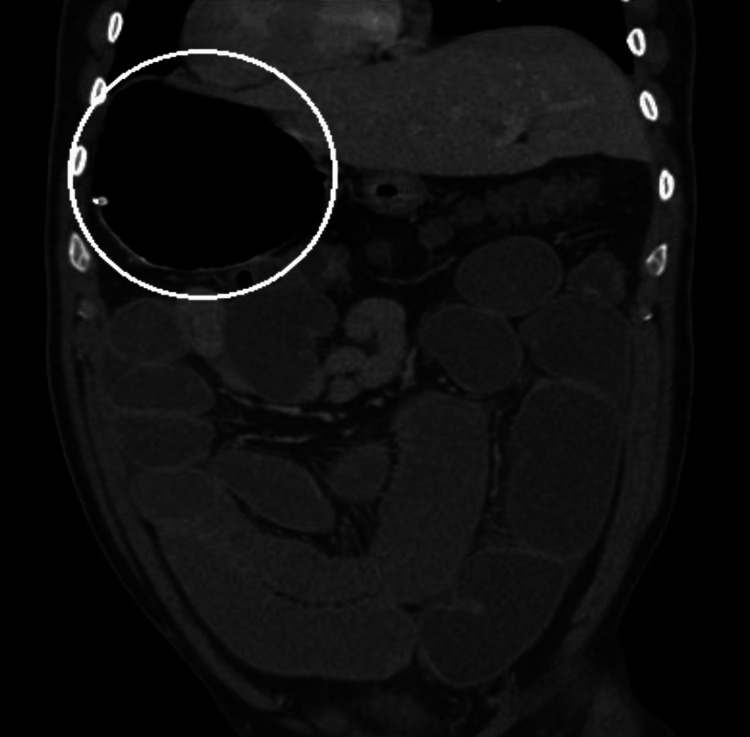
Coronal contrast-enhanced CT image demonstrating a right-sided distended stomach, consistent with mirror-image visceral orientation in situs inversus totalis, with associated dilated small-bowel loops in the mid-abdomen.
